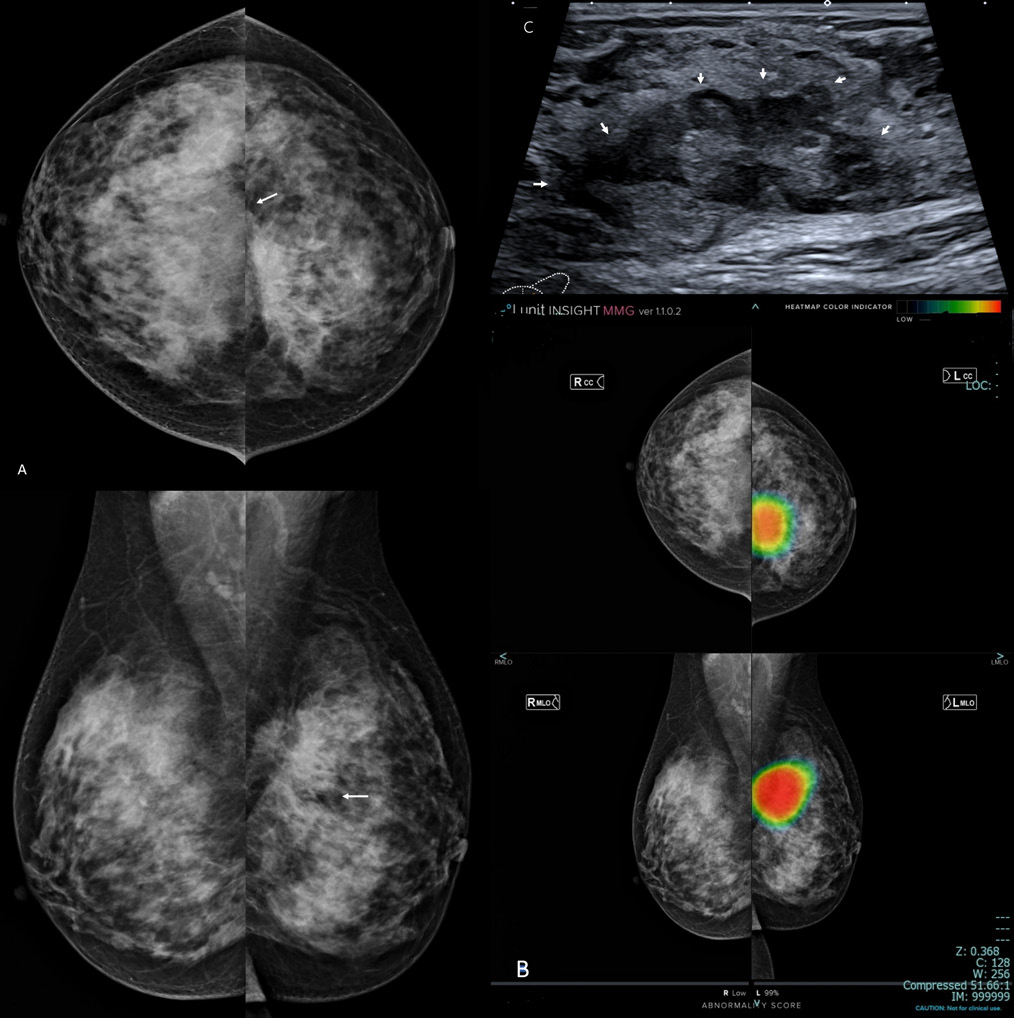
Left breast invasive ductal carcinoma grade III in a female patient 37-years-old with extremely dense breasts (ACR d). A: bilateral digital mammogram (cranio-caudal and medio-lateral oblique views). The density of the breasts decreased the mammogram sensitivity- there is no obvious masses at first sight- the left breast showed upper inner tissue peaking (arrow) which is indirect suspicion sign of malignancy. B: AI scanned mammogram presented intense color hue that targeted specifically the left breast mass, confirmed the suspicion of malignancy in the form of abnormality scoring of 99% and displayed the full extent of the disease. C: Ultrasound image displayed comparable information to that of the AI where there was 11 clock left breast purely solid irregular mass (BI-RADS 5) (arrows). The case was a true positive on the level of conventional imaging and AI algorithm
